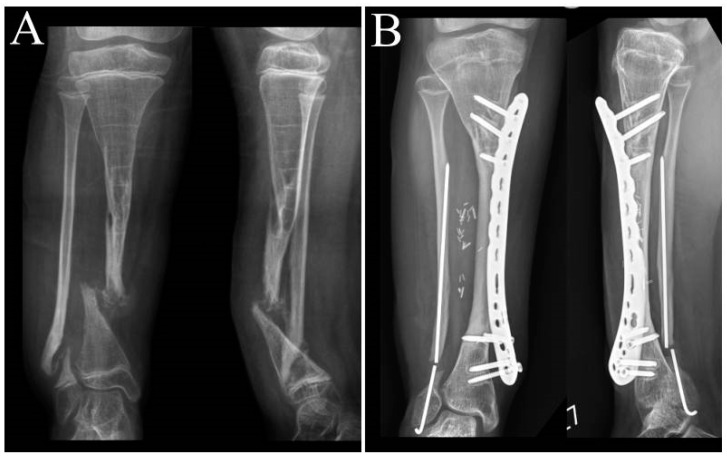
Radiographs of patient 1: (A) preoperative AP and lateral radiographs and (B) postoperative AP and lateral radiographs at 53 months after the surgery.
